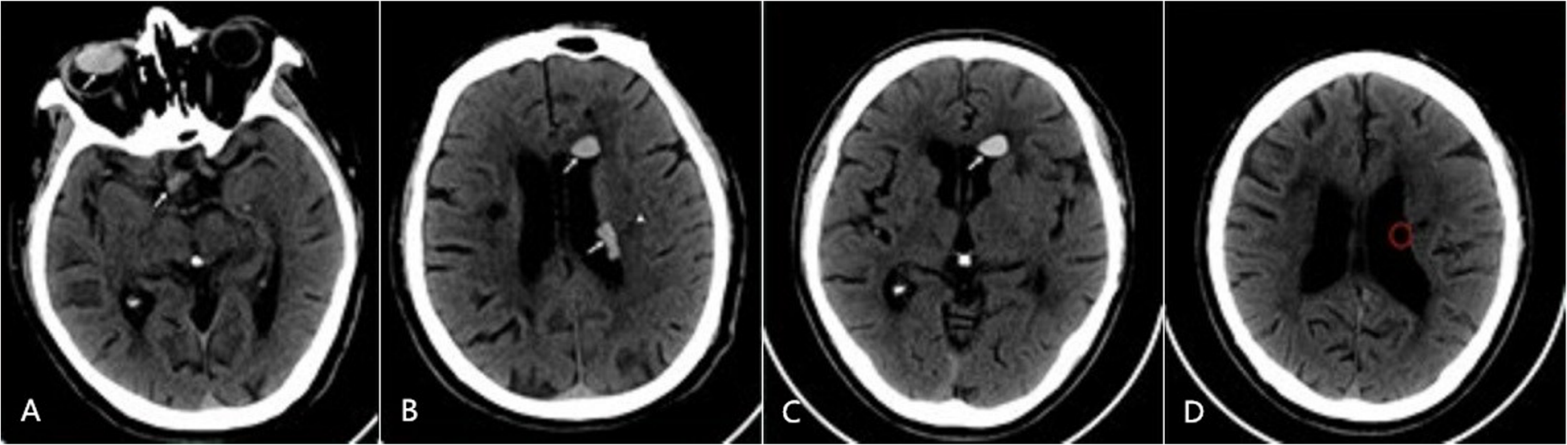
The patient undergoes CT examinations in March and June 2018. a, the high or slightly high density shadows in the right eyeball and the right suprasellar cistern (ww 60, wl 100)(arrow); b, the high density shadow at anterior horn and middle of the left lateral ventricle (ww/wl same as A)(arrow), the slightly low density shadow at the left corona radiata (arrowed), prompting infarction (subsequently confirmed by MRI); c~d, CT reexamination after 3 months shows that the lesion at the left lateral ventricular anterior horn has a higher density and a larger volume (ww/wl same as A) (arrow), but it disappears in the middle of left lateral ventricle (ww/wl same as A)(red circle)
